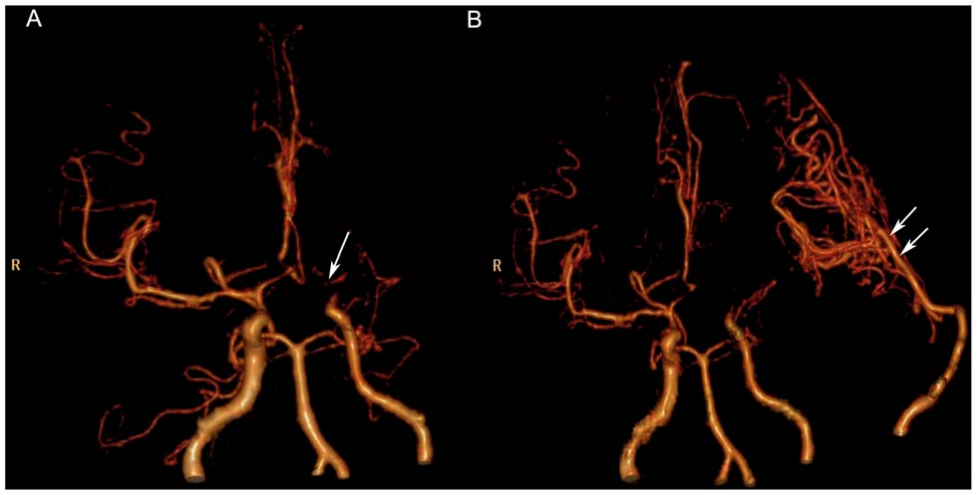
CT Angiography (CTA) images in frontal projection before and after bypass in Moyamoya disease. (A) The preoperative volumetric CTA acquired in the arterial phase of whole-brain CT perfusion (CTP) shows the left internal carotid artery (ICA) is occluded in the supraclinoid portion, and “Moyamoya vessels”(arrow) can be seen in the cerebral base. The right supraclinoid ICA and the proximal anterior cerebral artery (ACA) and middle cerebral artery (MCA) are markedly stenosed. (B) The arrows indicate the left-side direct graft patency with collateral vessels from the left external carotid artery to the MCA territory on the postoperative volumetric CTA acquired in the arterial phase of whole-brain CTP.
